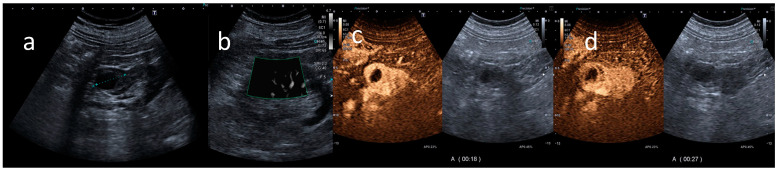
CEUS findings. B-mode ultrasound shows a complex cystic lesion irregularly thickened (a), with intravascular signal at SMI (b). In the arterial phase, after using a contrast agent (Sonovue), the contrast-enhanced ultrasound shows a marked enhancement (c) and a rapid wash-out (d) of the wall and of solid nodular components of the wall, with an un-enhanced central core of necrosis. These findings were assessed by the radiologist as positive findings for malignant small renal mass. Final pathologic assessment confirmed this assessment, as it was diagnosed as a renal cell carcinoma with foci of necrosis.
